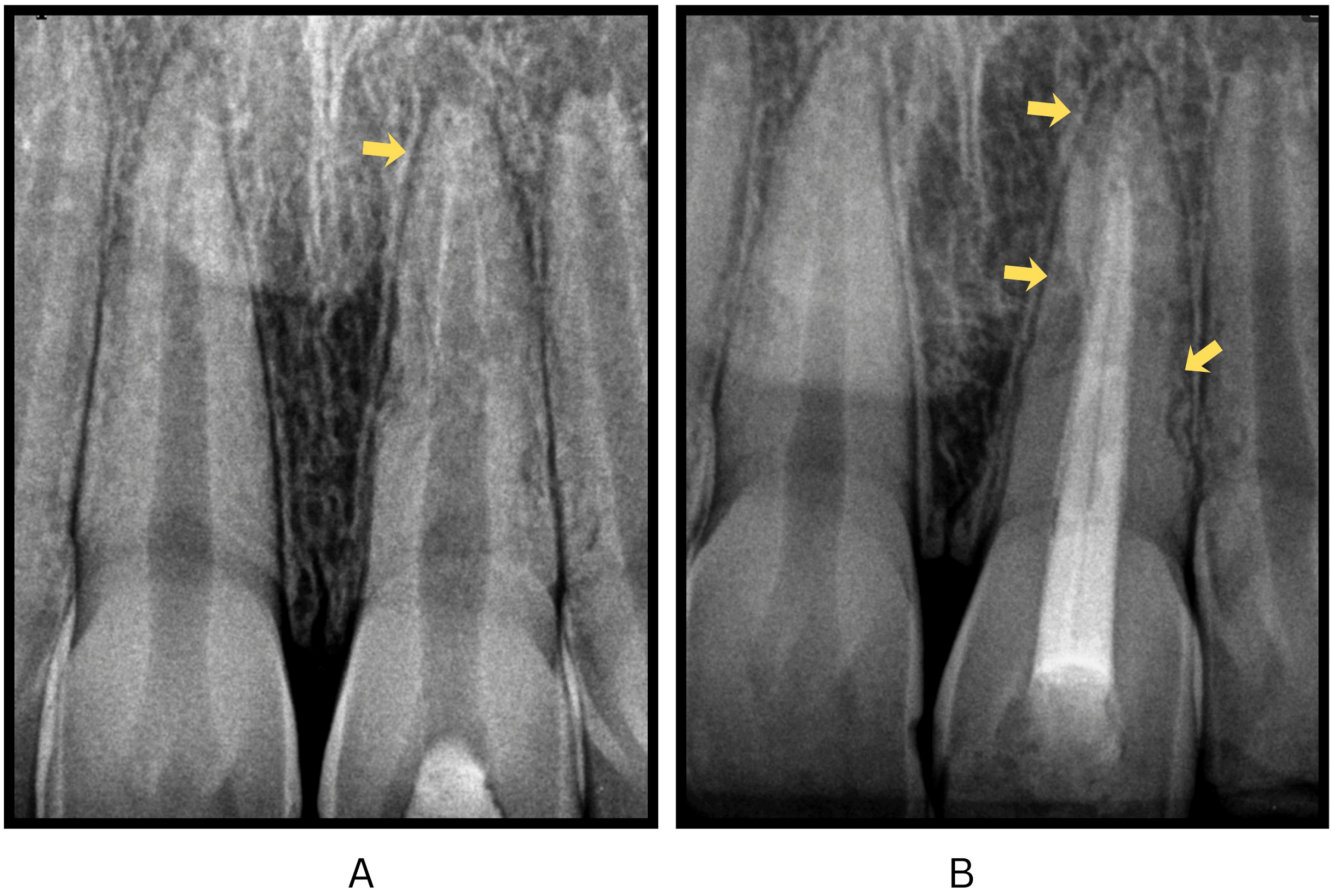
Periapical radiographs demonstrating MTA apexification and subsequent obturation of the replanted maxillary left central incisor. (A) Mineral trioxide aggregate (MTA) apexification (marked by arrow) performed during the three-year follow-up using the apical barrier technique in #21. (B) Intraoral periapical radiograph following obturation of tooth #21, showing successful apical closure and evidence of satisfactory periapical healing (marked by arrows).
